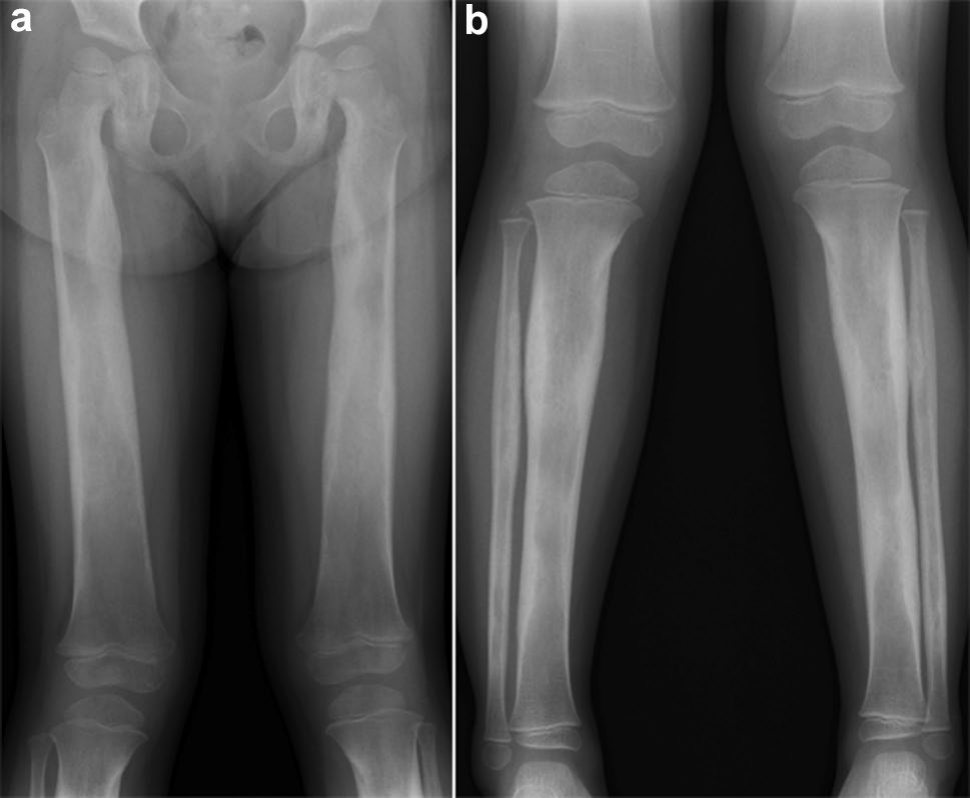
a, b A 3-year-old girl with diaphyseal dysplasia (Camurati–Engelmann disease). Radiograph shows cortical thickening with coinciding medullary narrowing of the long bones
